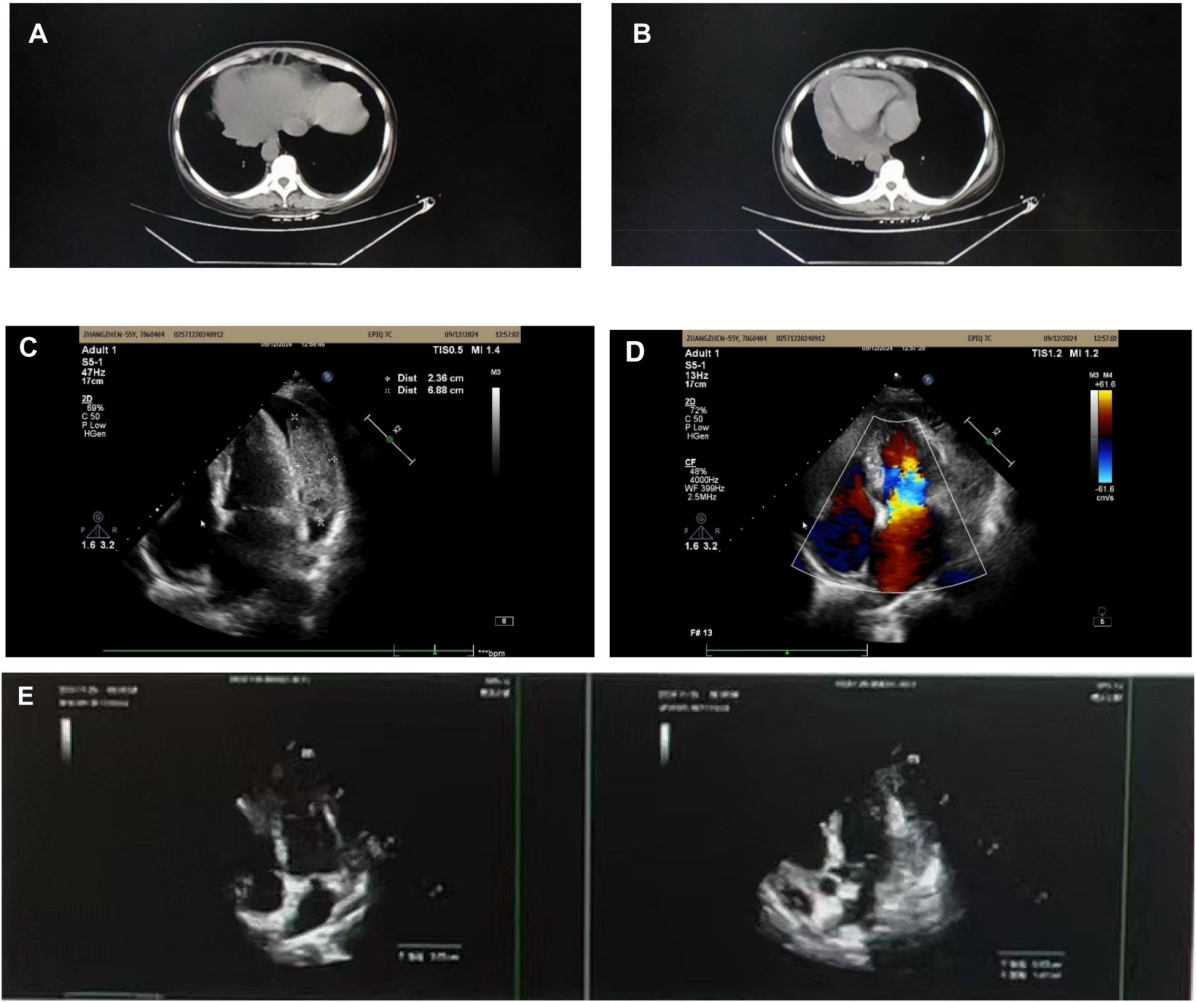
(A) Bilateral pleural effusion. (B) Pericardial effusion. (C,D) Pericardial effusion with multiple masses. (E) 6-month follow-up echocardiogram.
